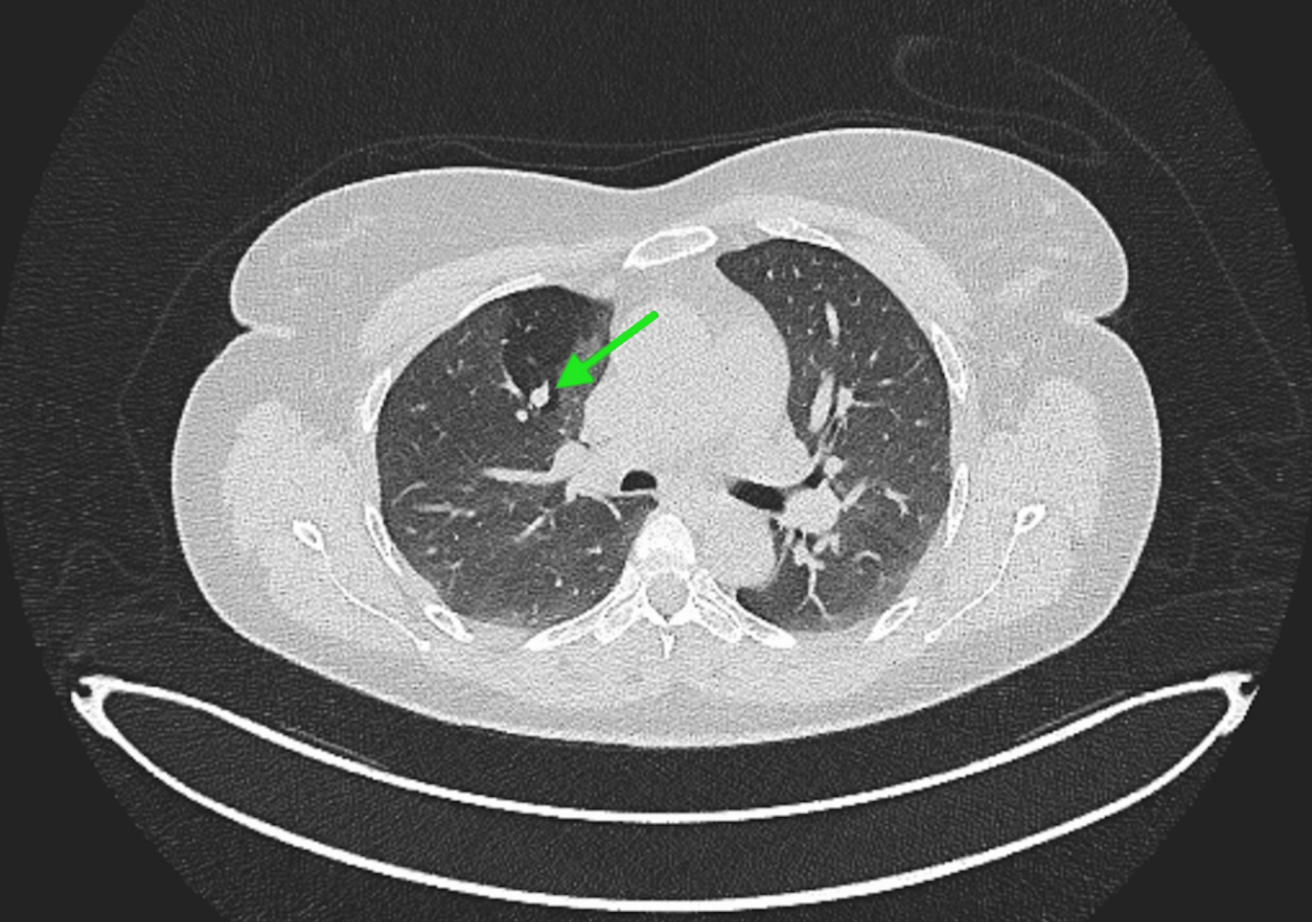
CT scan showing suspicion of lung cancer in the right lung.
